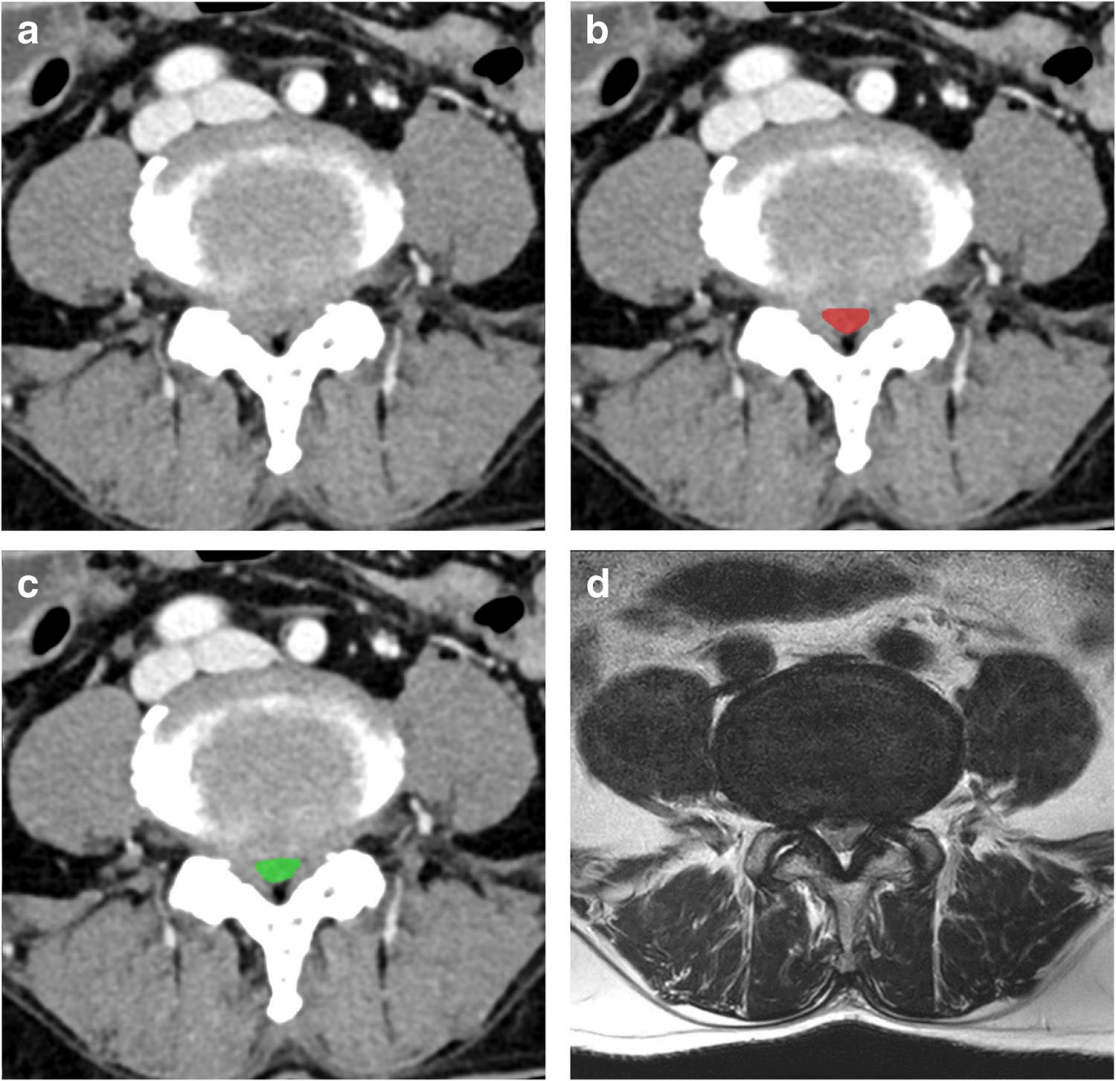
Representative cases of dural sac segmentation on a stenosis case with contrast on abdominal CT. The images of axial enhanced abdominal CT imaging (a) selected from the dataset are shown alongside their resulting segmentation of the dural sac by a manual rater (b, red) and the proposed algorithm (c, green). The dural sac area is 50.6 mm^2^ (b) and 45.6 mm^2^ (c), respectively. Figure 6d is an MR axial image of this case, which was taken within 6 months of the CT scan
